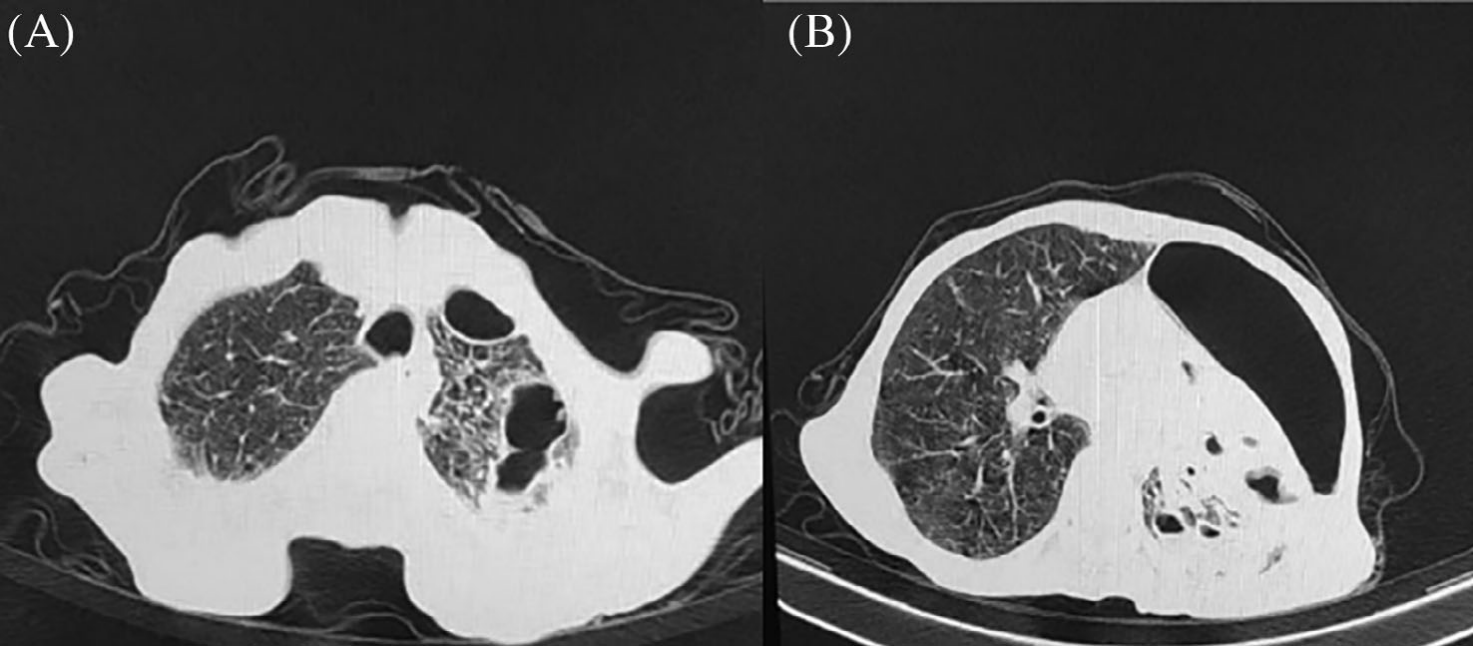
(A) Cavities in the left upper lobe (an axial section of the chest computed tomography [CT] scan) and (B) pneumothorax in the left upper lobe (an axial section of the chest CT scan)
